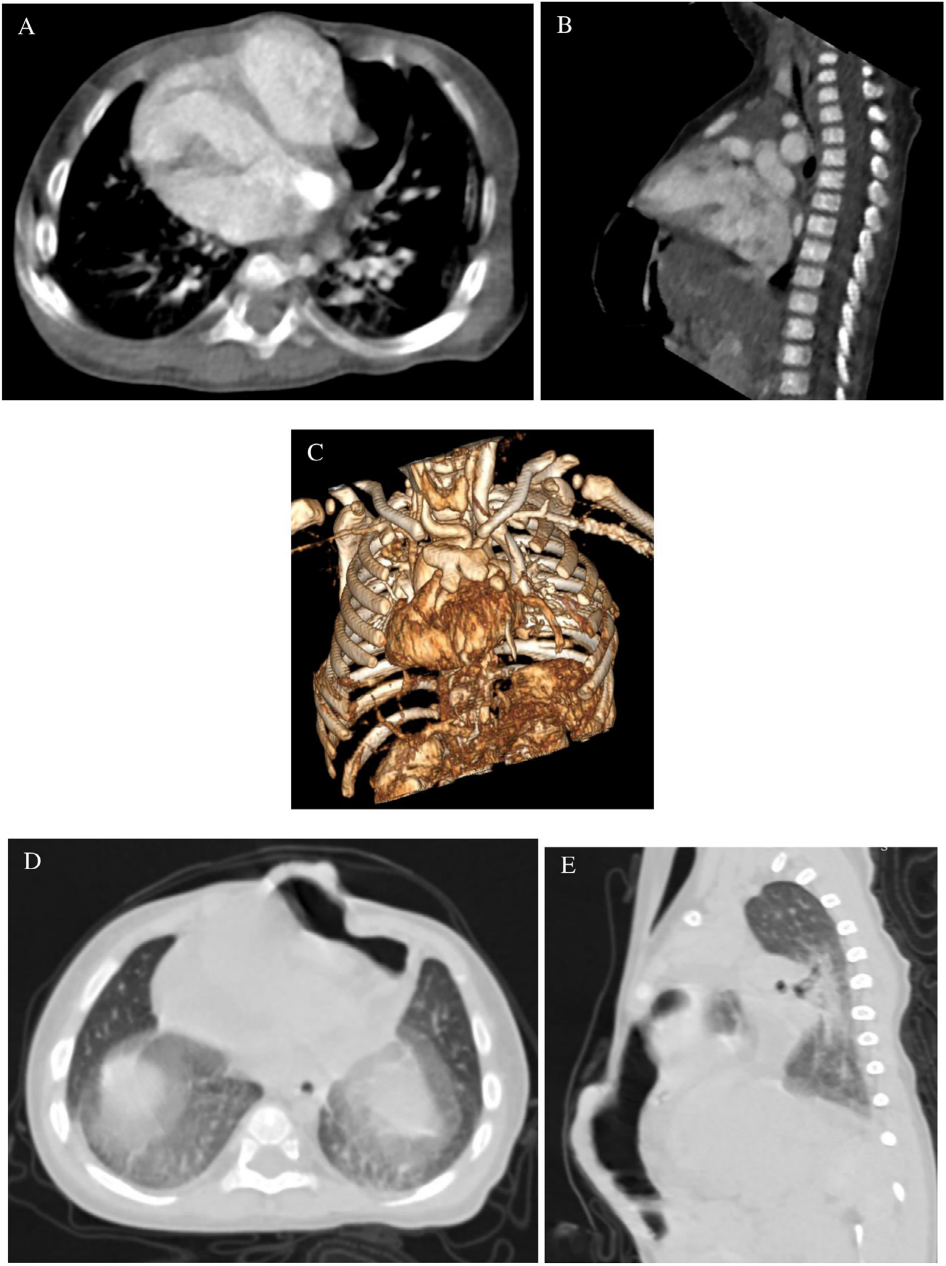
Axial (A) and sagittal (B) chest computed tomography (CT) scans showed a defect of the sternum (red arrow) and a herniated left ventricle of the heart. Bone 3-dimensional (3D) reconstruction (C) showed a defect in the sternum. Axial (D) and sagittal (E) upper abdominal CT scans revealed a defect of the anterior diaphragm, with a hernia in the transverse colon through the defect and up to the subcutaneous layer.
